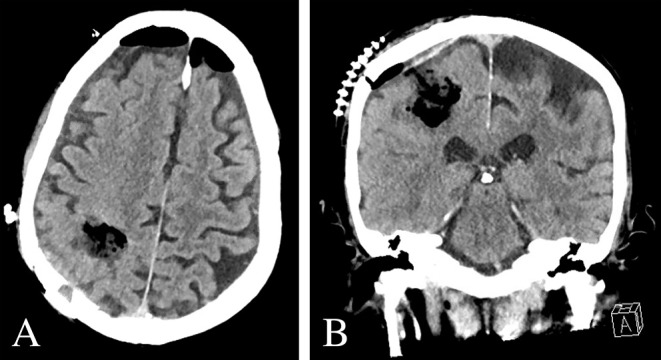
Post-operative imaging. (A,B) Post-operative CT Head demonstrated expected post-operative changes without evidence of acute pathology.
